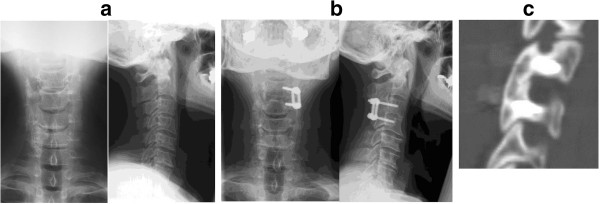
Unilateral pedicle screw fixation (patient number 7). (a) Plain radiography of the cervical spine of a 60-year-old man showed a positive pedicle sign at the left C4. (b) Plain radiography at the last follow-up showed a good union with no implant failure at the last follow-up. (c) Sagittal CT at the last follow-up.
